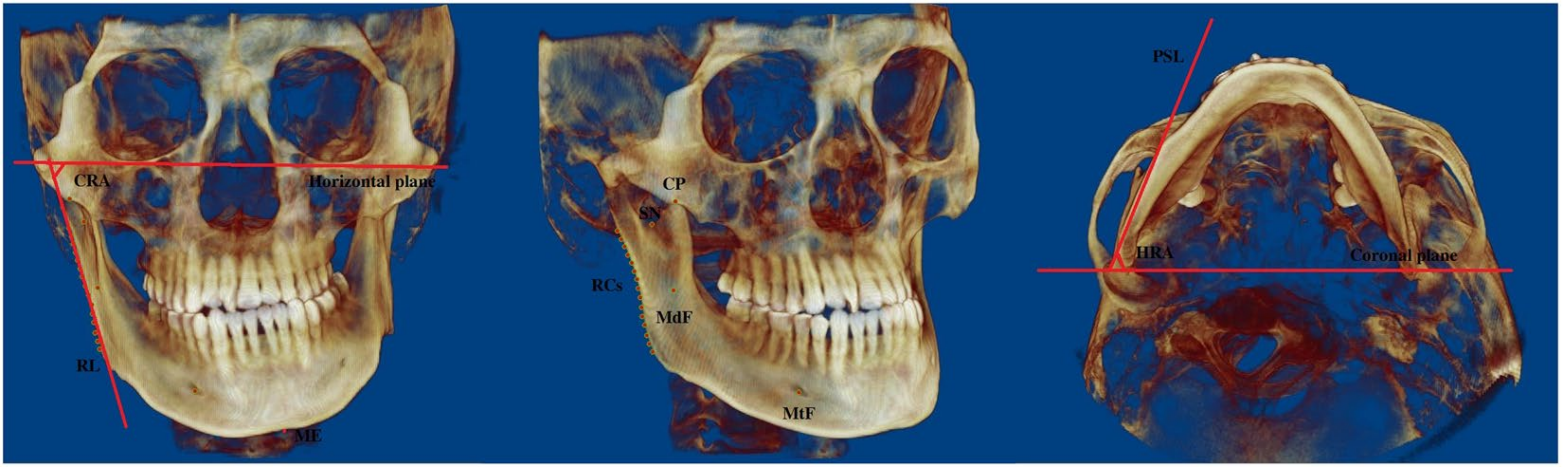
Landmarks and reference lines for 3D-CBCT evaluation. SN: sigmoid notch; CP: Coronoid process; MdF: Mandibular foramen; MtF: Mental foramen; RCs: Ramus contour point; Me: Menton; RL: Ramus line; CRA: Coronal ramus angle. PSL: Proximal segment line, the line connecting CP and SN projected no to horizontal plane; HRA: Horizontal ramus angle.
